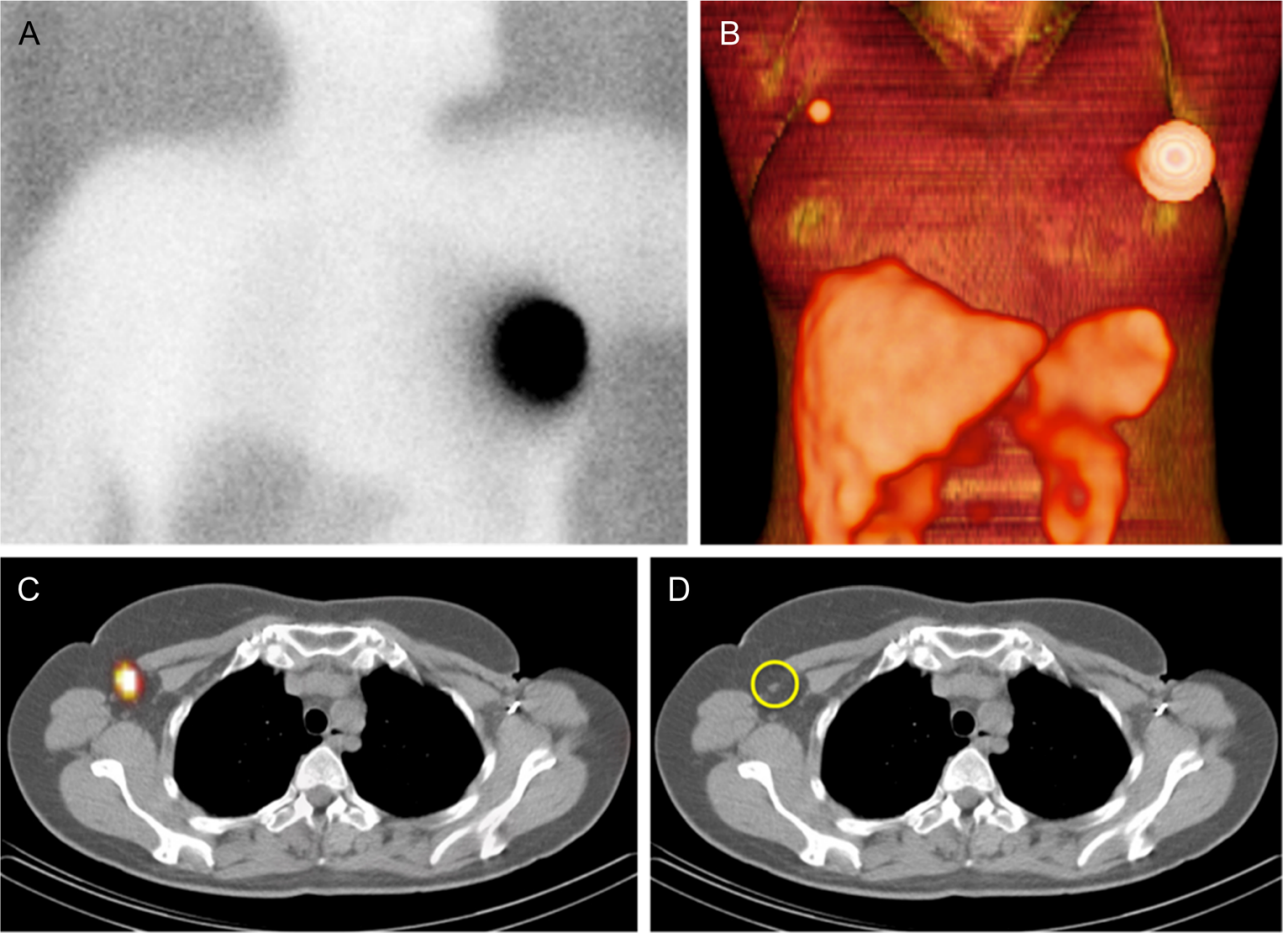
A 48-year-old woman with a ductal carcinoma relapse in the left breast, without SN visualization on planar image (a). Volume rendering of SPECT/CT (b) shows a focus in level 1 of the contralateral axilla (figure c) corresponding with a non-enlarged lymphatic node (circle) on CT (d)
